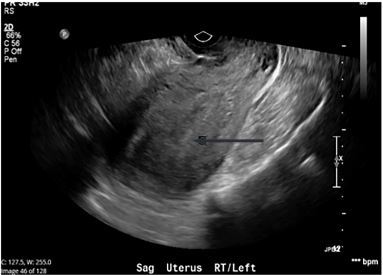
Transvaginal ultrasound showing thickened endometrium (arrow) with no intrauterine pregnancy.
